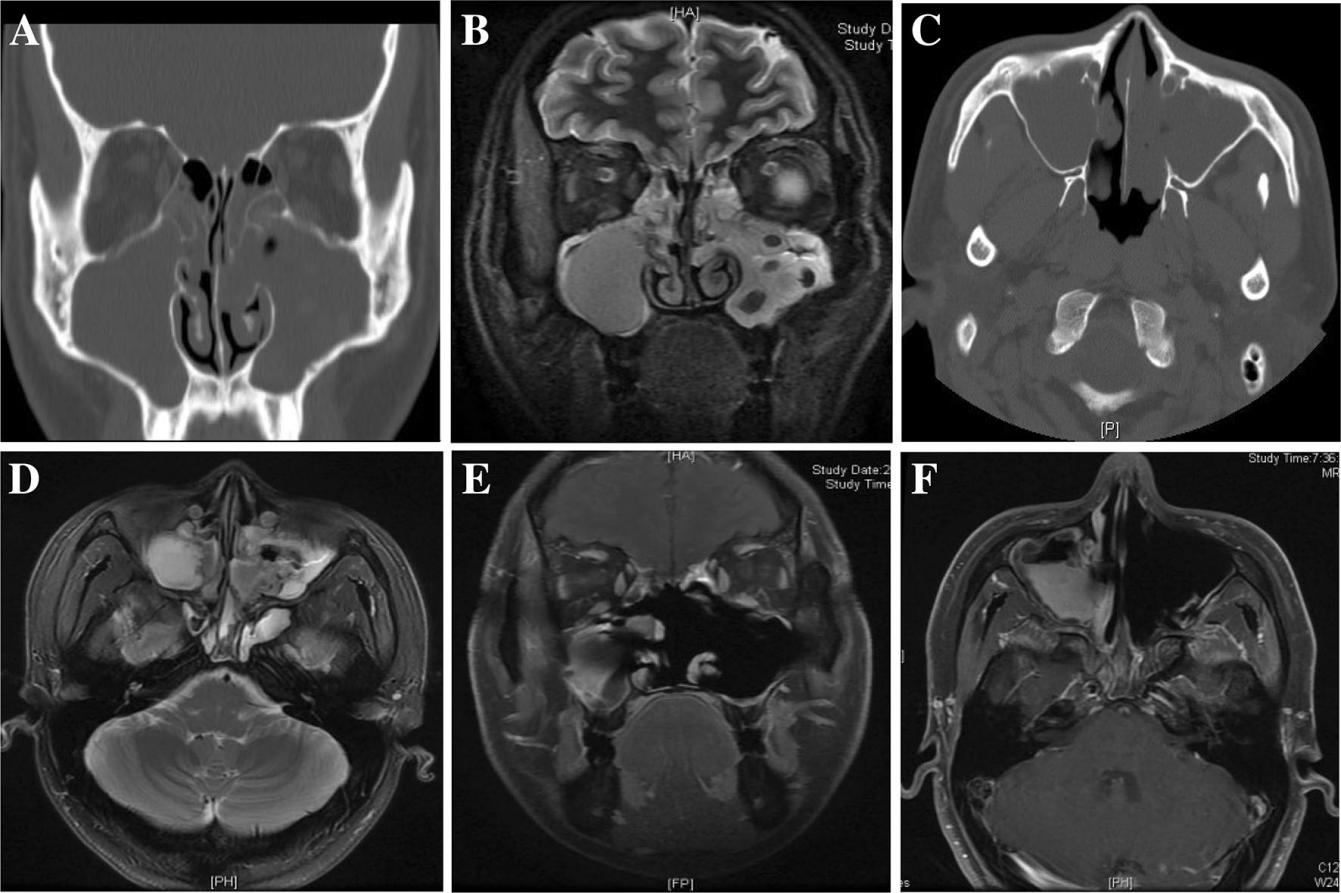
Preoperative and postoperative paranasal sinus CT and MRI of IP of maxillary sinus. a Coronal CT demonstrated opacification of the bilateral maxillary sinus. b Coronal T2-weighted MR image with contrast showed IP as an intermediate and irregular mixed signal intensity mass of the left-side maxillary sinus. c Axial CT demonstrated opacification of the bilateral maxillary sinus. d Axial T2-weighted MR image showed the tumor lying around the left-side maxillary sinus. e Postoperative coronal MRI showed a clear left and right maxillary sinus with thickened mucosa and no tumor recurrence. f Postoperative axial MRI showed a clear left and right maxillary sinus with thickened mucosa and no tumor recurrence
